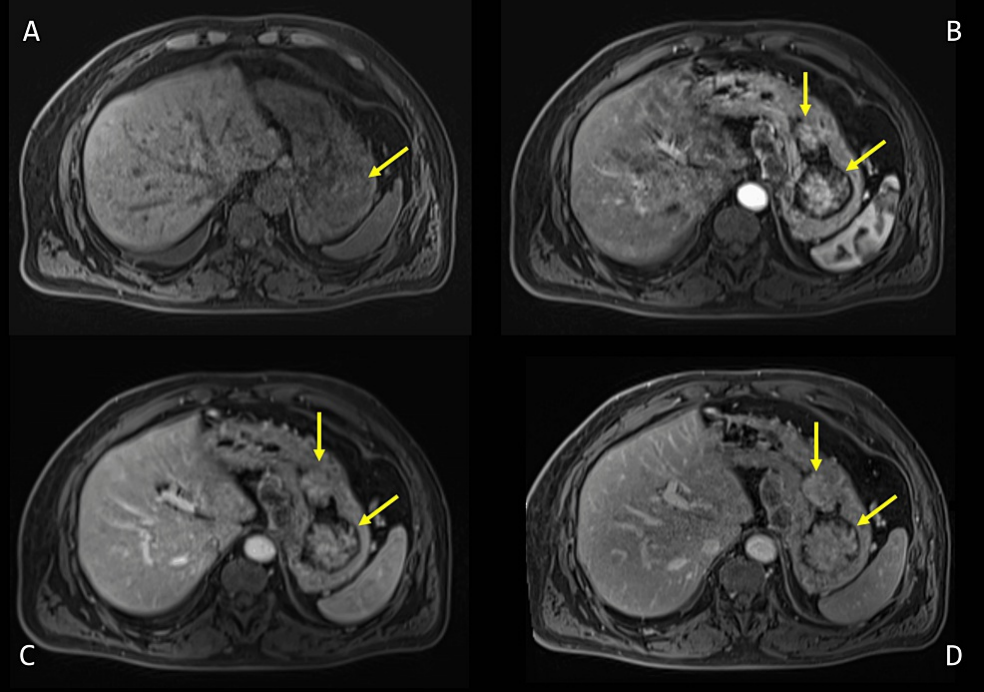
Axial fat-saturated unenhanced T1-weighted (A) and axial fat-saturated contrast-enhanced T1-weighted magnetic resonance imaging of the abdomen on arterial phase (B), portal phase (C) and three-minute late venous phase (D) The gastric lesions (yellow arrows) appear hypointense on the fat-saturated T1-weighted image (A). They exhibit the characteristic hypervascular behavior of clear cell renal cell carcinoma, demonstrating intense early heterogeneous enhancement during the arterial phase (B), progressing during the portal venous phase (C) and the three-minute late venous phase (D).
